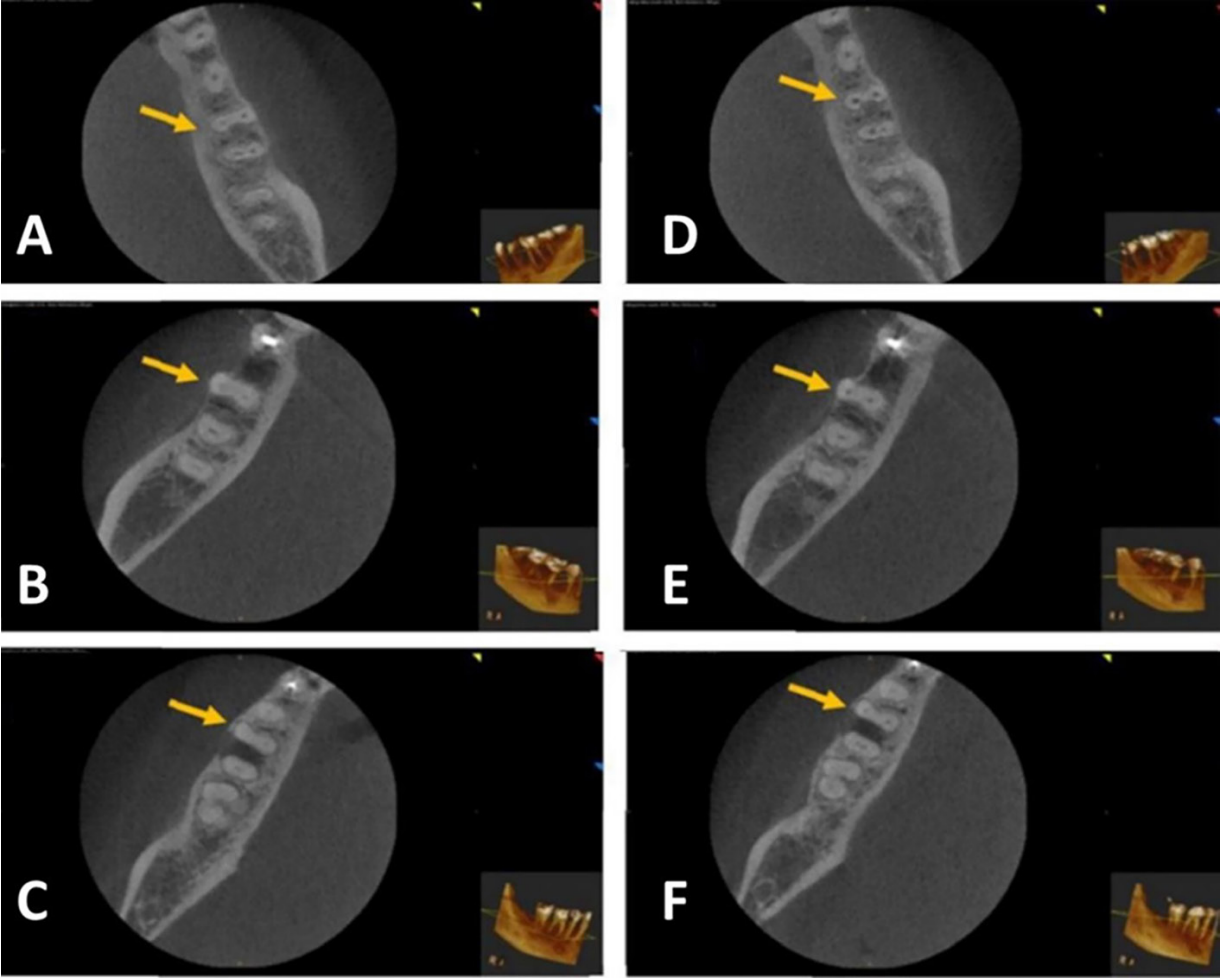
CBCT images for pre-instrumentation (A, B, C) and post-instrumentation (D, E, F) with Tornado (A, D), WaveOne (B, E), and OneShape systems (C, F) at 8 mm from the apex.
